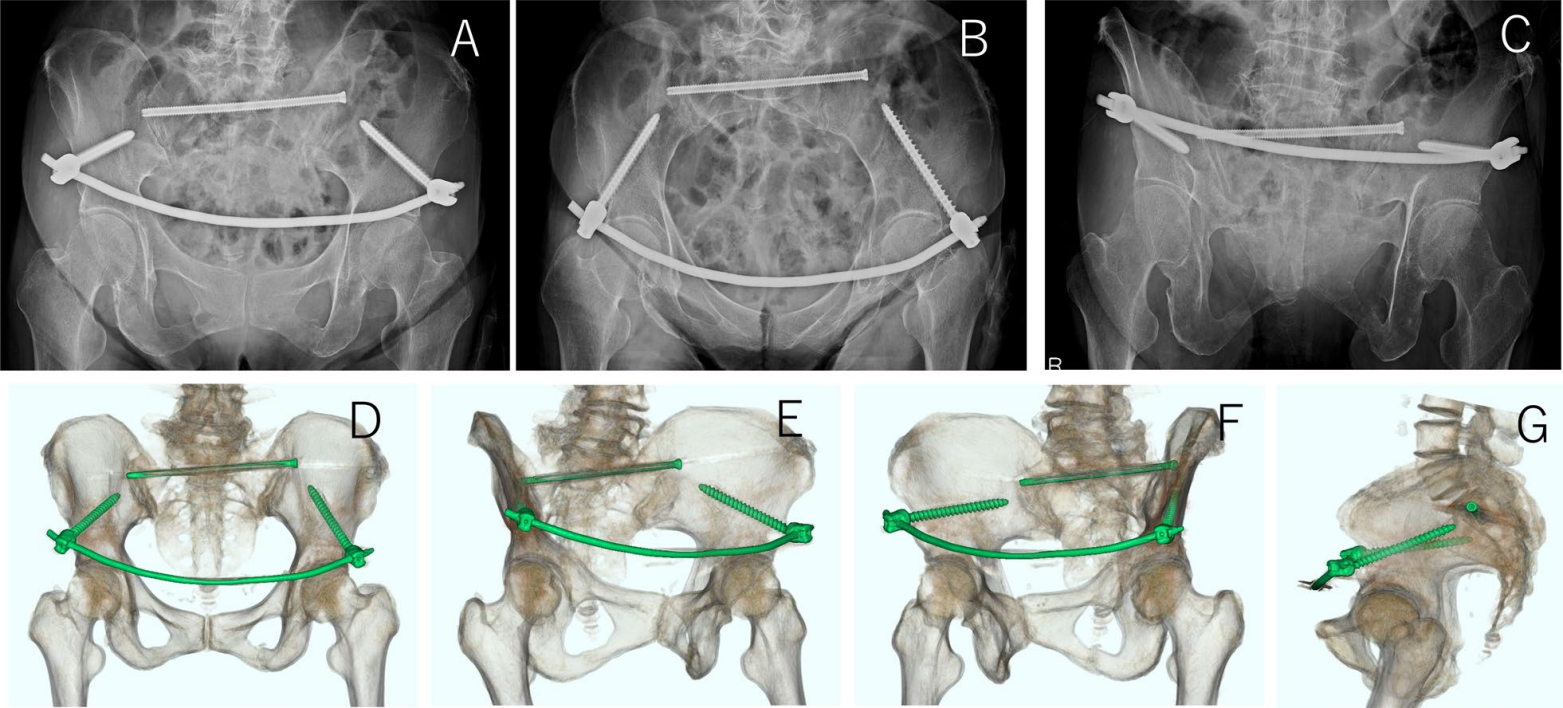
Postoperative X-rays and CT. X-rays; Pelvic AP view (a), Pelvic inlet view (b), and Pelvic outlet view (c). Postoperative three-dimensional CT reconstruction; AP view (d), Obturator oblique view (e–f), and lateral view (g)
